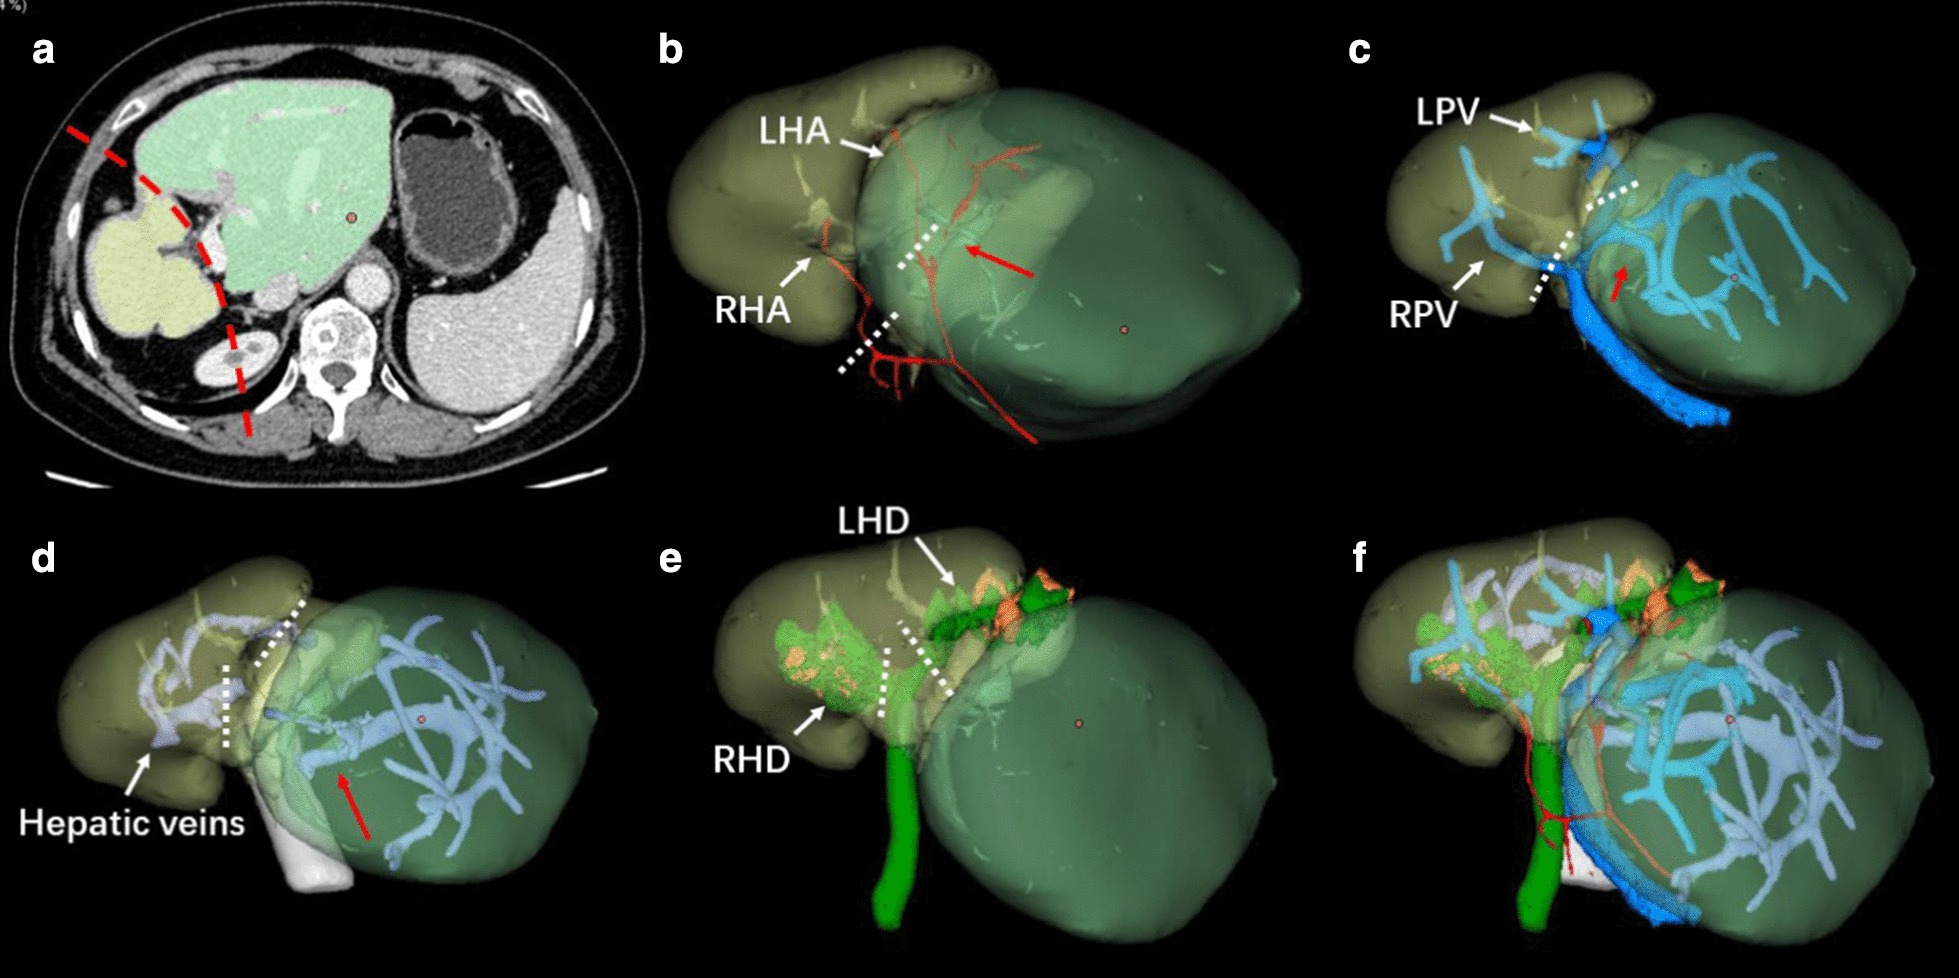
3D liver reconstruction of case 1. a Reflection of 3D reconstruction in CT image. Green part: hypertrophic caudate lobe; Yellow part: atrophic main liver; Dashed red line: boundary of caudate lobe and main liver. b Reconstruction of hepatic arteries. Dashed white line: transection of hepatic arteries; Solid red arrow: hepatic artery of caudate lobe. c Reconstruction of portal veins. Dashed white line: transection of portal veins; Solid red arrow: portal vein of caudate lobe. d Reconstruction of hepatic veins. Dashed white line: transection of hepatic veins; Solid red arrow: short hepatic veins. e Reconstruction of bile ducts. LHD and RHD were filled with stones. Dashed white line: transection of hepatic ducts. f Configuration of the vasculatures. *LHA* left hepatic artery, *RHA* right hepatic artery, *LPV* left portal vein, *RPV* right portal vein, *LHD* left hepatic duct, *RHD* right hepatic duct
